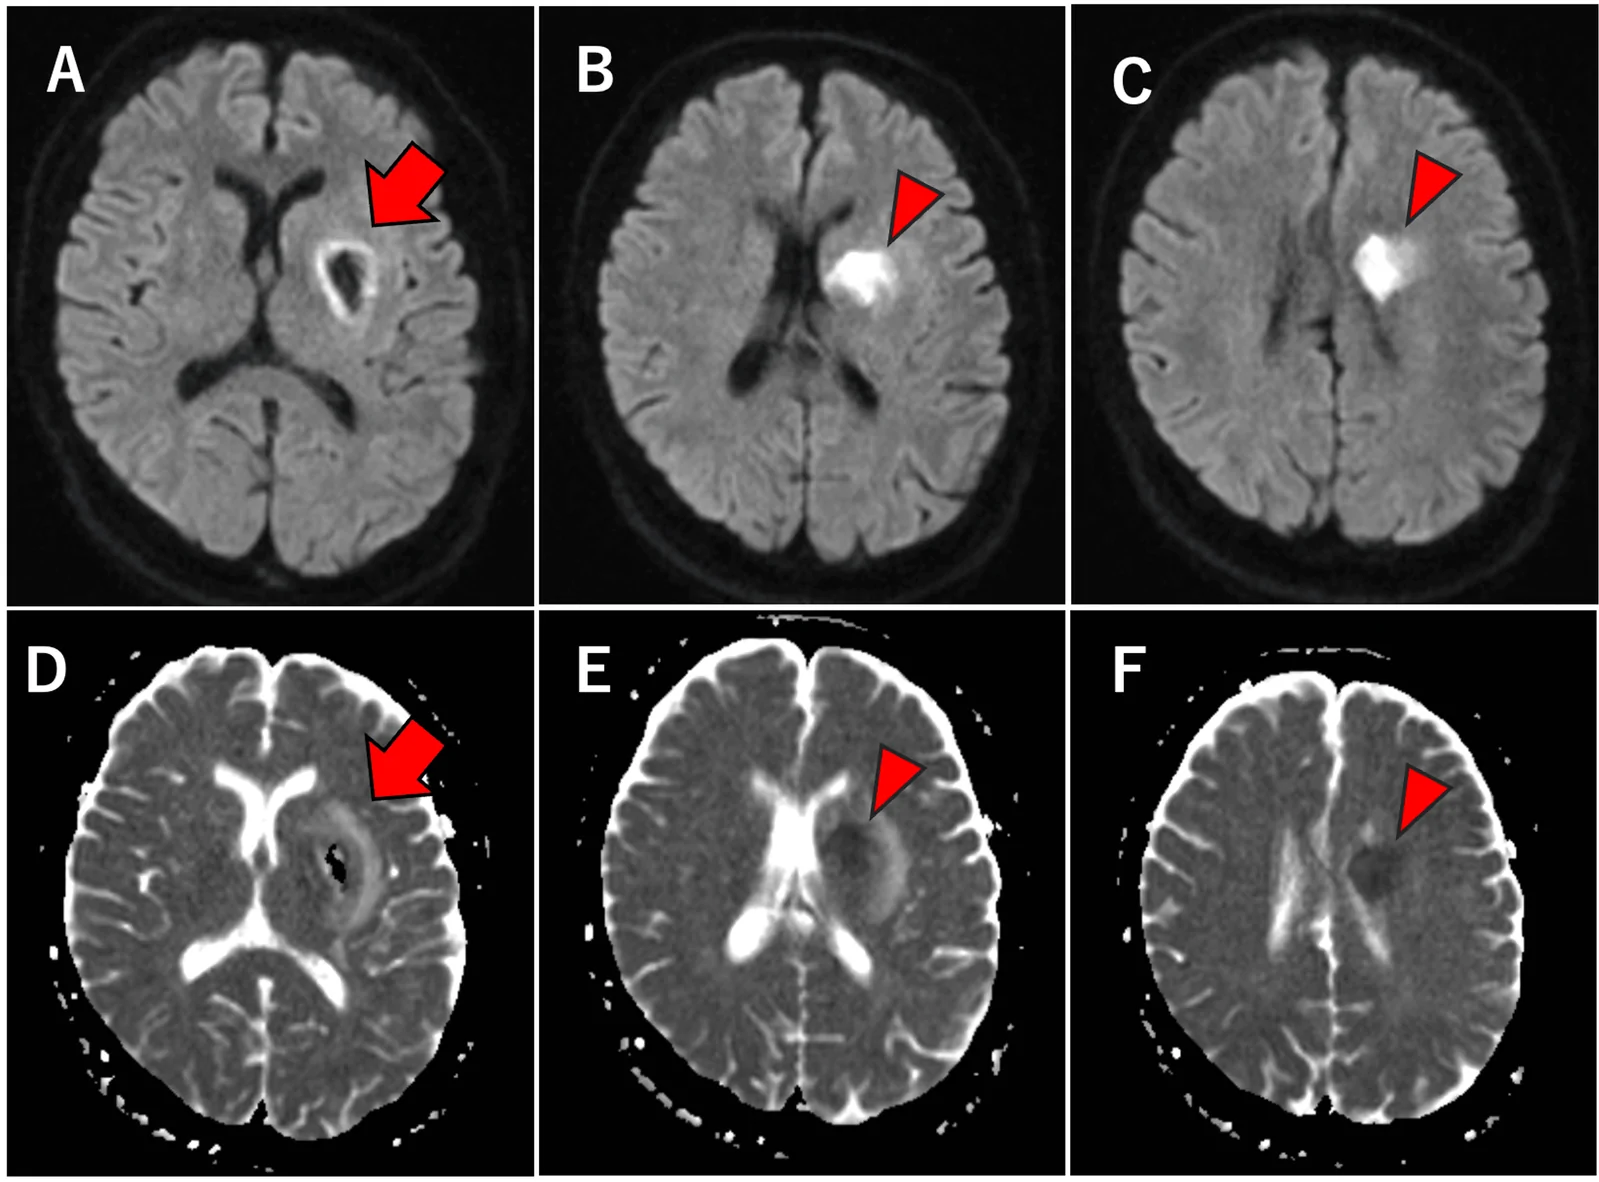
Magnetic resonance imaging findings. (A) Axial diffusion-weighted imaging showed a left putaminal hematoma characterized by a central area of low signal intensity - due to magnetic susceptibility effects - surrounded by a hyperintense rim of perihematomal edema (arrow). (B, C) More cranial slices demonstrated a hyperintense lesion adjacent to the superomedial aspect of the hematoma, extending toward the left corona radiata within the lenticulostriate artery territory (arrowheads). The lesion was consistent with acute infarction. (D, E, F) Corresponding apparent diffusion coefficient maps showed high signal intensity in the perihematomal region, consistent with edema (arrow), whereas the adjacent lesion extending toward the corona radiata showed low signal intensity, indicating restricted diffusion and acute infarction (arrowheads).
